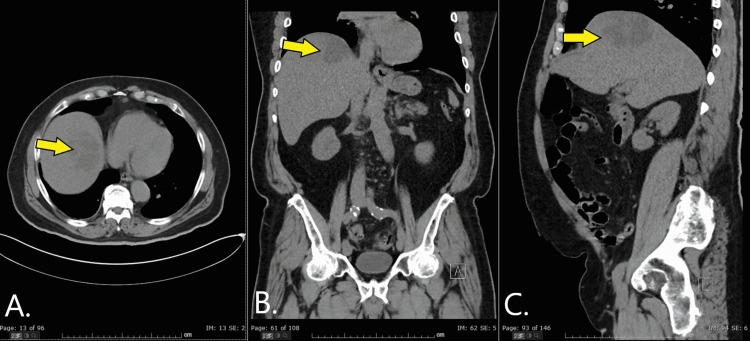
Axial (A), coronal (B), and sagittal views of CT scan abdomen/pelvis without contrast The images show an approximate 8 cm lesion (yellow arrow) in the superior portion of the right hepatic lobe.
